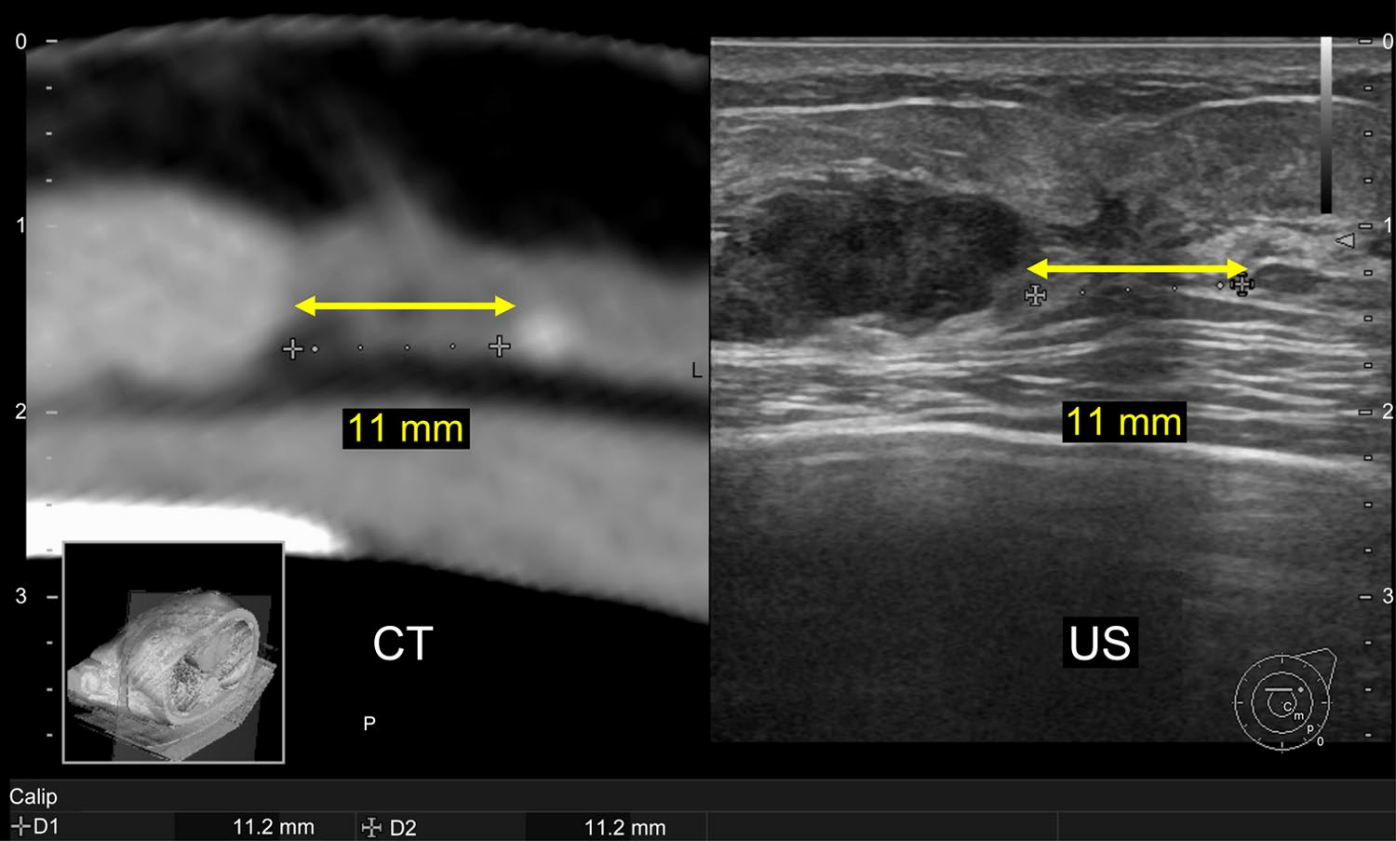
CT/ultrasound fusion image. As contrast CT scans are performed in the same position as ultrasound, the images are easily fused. In this figure, the distance between the lesions in both the CT and ultrasound images is 11 mm
